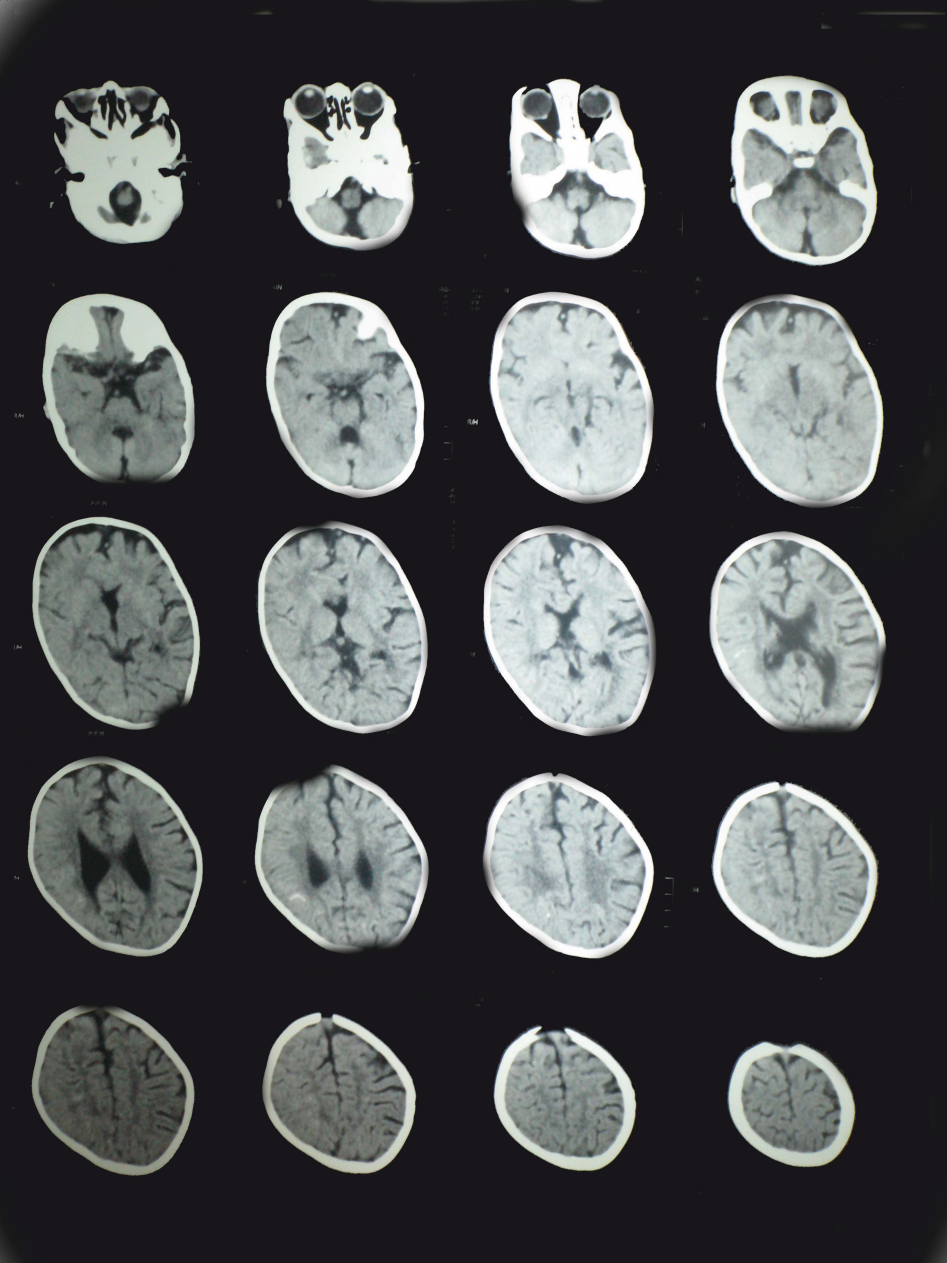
Computed tomography of her brain showing holoprosencephaly with absent corpus callosum.
